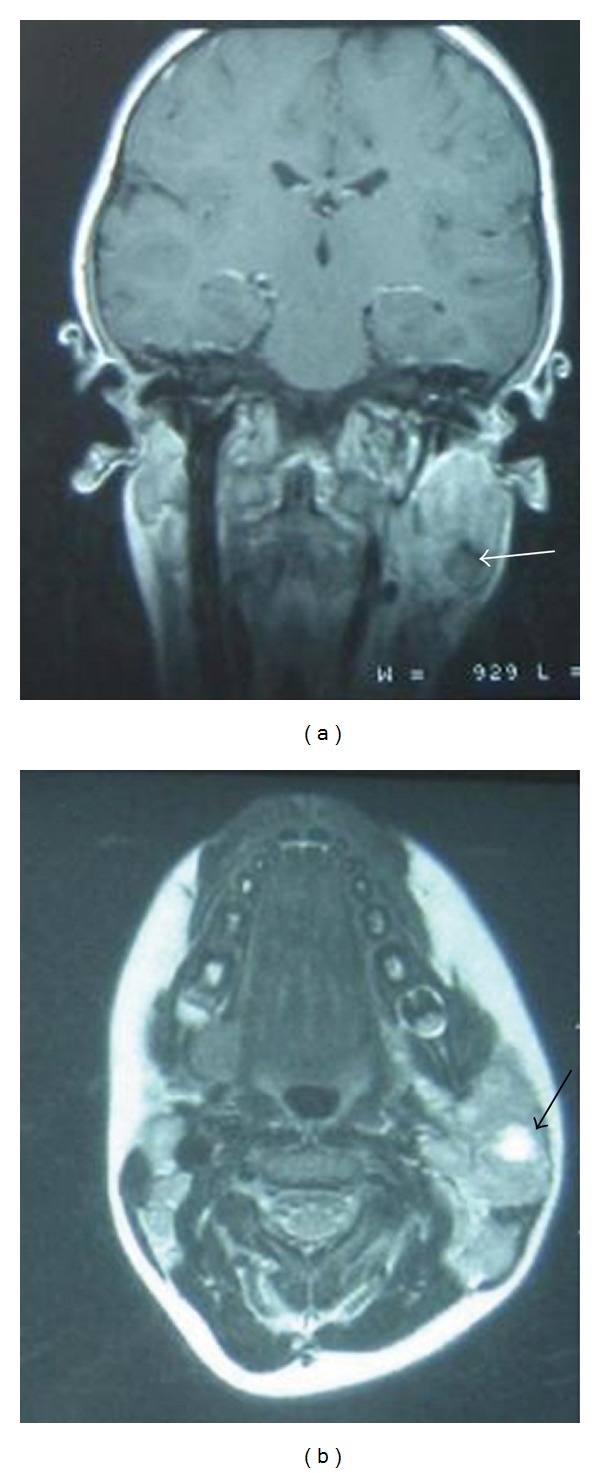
Coronal T1-weighted (a) and axial T2-weighted (b) cervical magnetic resonance imaging showing a parotid gland tumor with a heterogeneous signals with a central area of necrosis (arrow).
